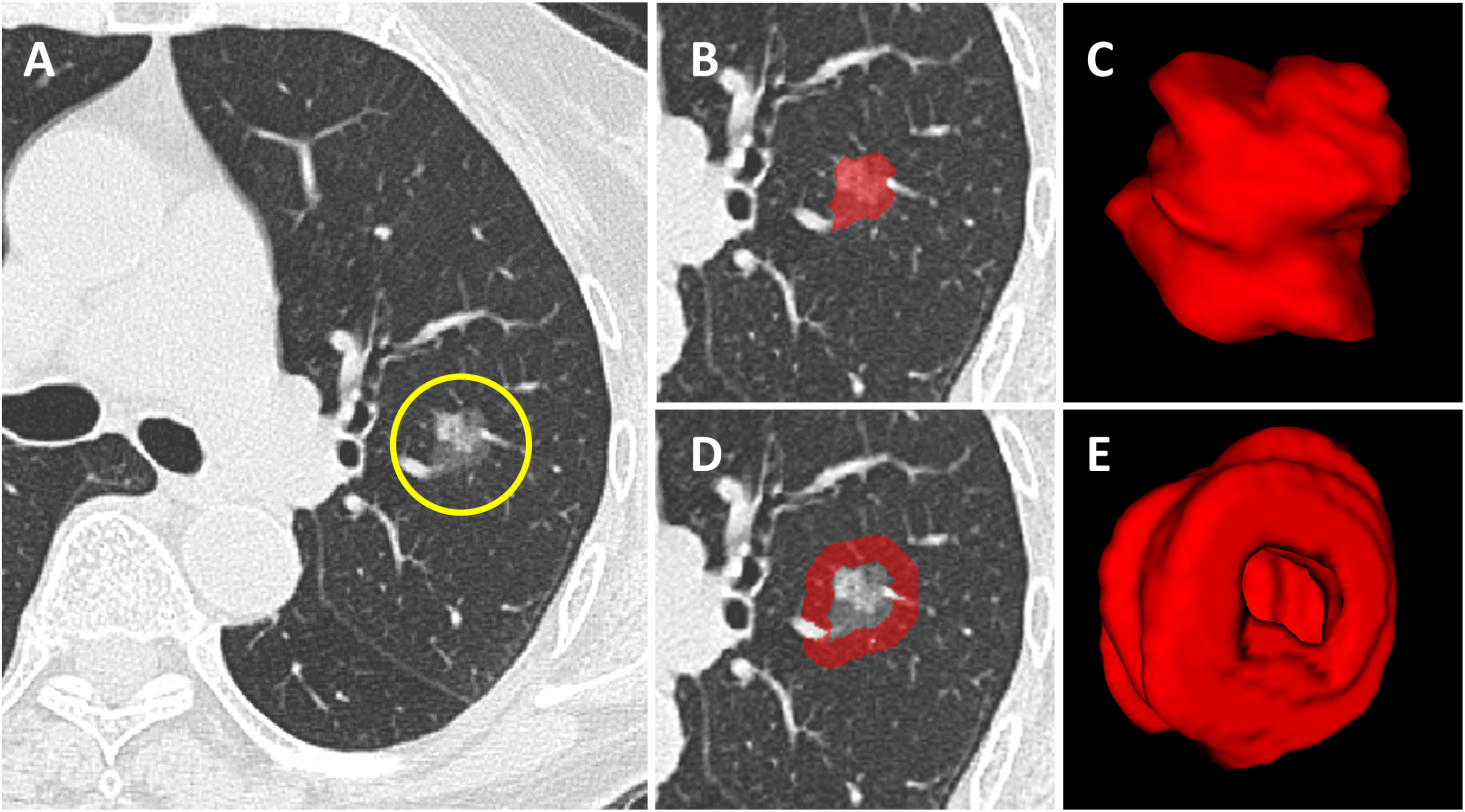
Tumoral and peritumoral area segmentation. (A) Computerized tomography (CT) image of a subsolid lesion pathologically confirmed as invasive lung adenocarcinoma. (B) The region of interest of the tumor is manually outlined layer by layer along the inner edge of the tumor. (C) The constructed 3D model of the intratumoral volume in ITK-SNAP. (D) The image showing the peritumoral region based on auto-dilating segmentation program, large blood vessels around the tumor are all excluded. (E) The constructed 3D model of the peritumoral volume in ITK-SNAP.
